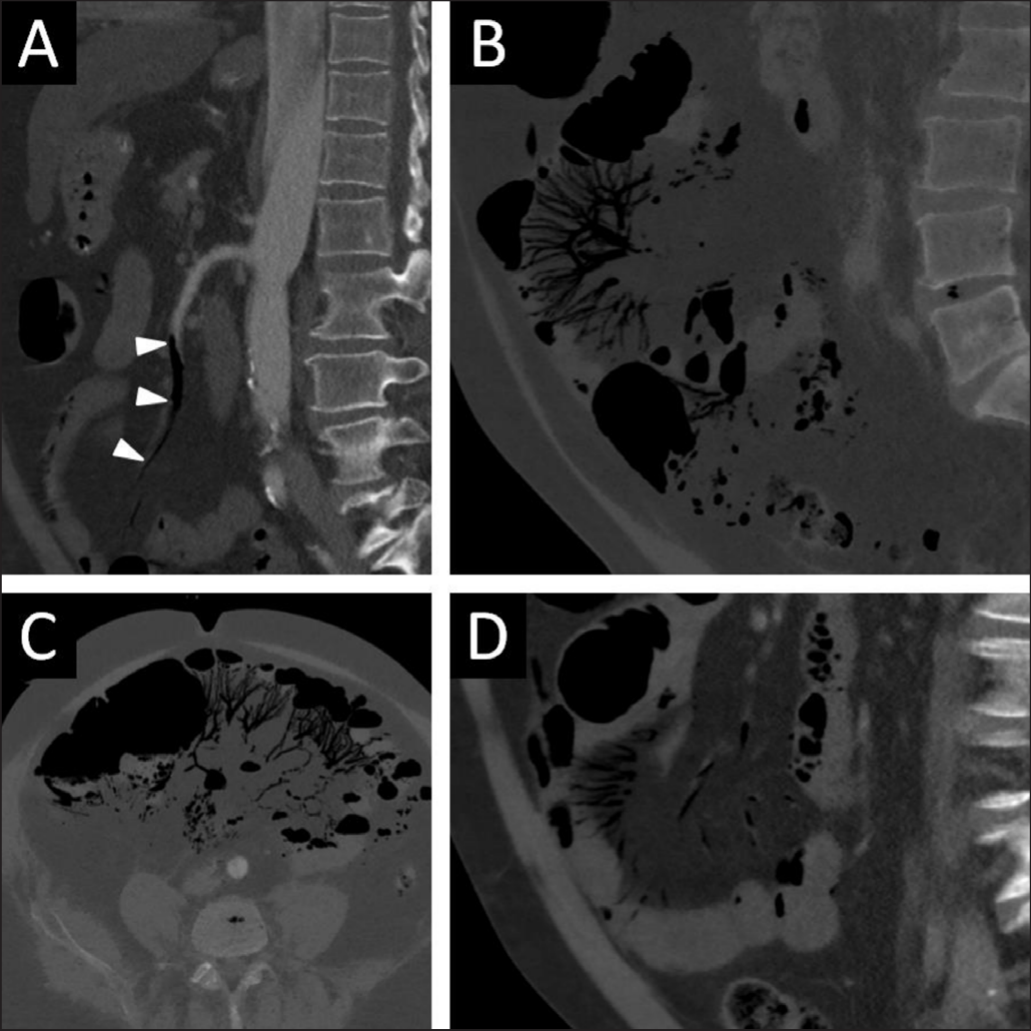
Gas bubbles in the superior mesenteric artery (a) and its branches extending up to the arcades (b) shown on the early CT on the first postoperative day. Small bowel loops remain without significant distension and contain only a small amount of fluid. A second CT on the first postoperative day (c, d) 14 hours later shows that the gas bubbles migrated further into the periphery of the branches of the superior mesenteric artery and into the bowel wall.
